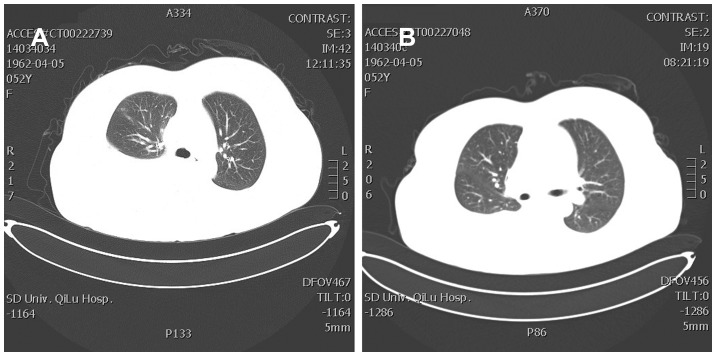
Chest computed tomography: comparison of pre- and post-operation. (A) Prior to operation and (B) seventh day post-operation.
